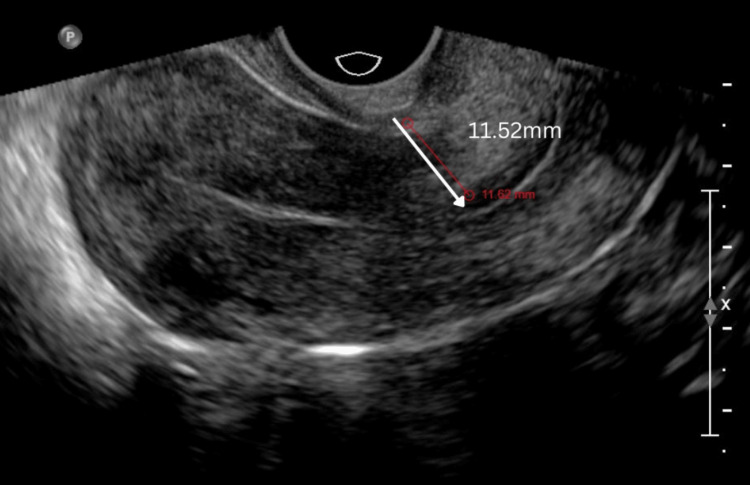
Postoperative ultrasonography with myometrial thickness of 11.52 mm.
